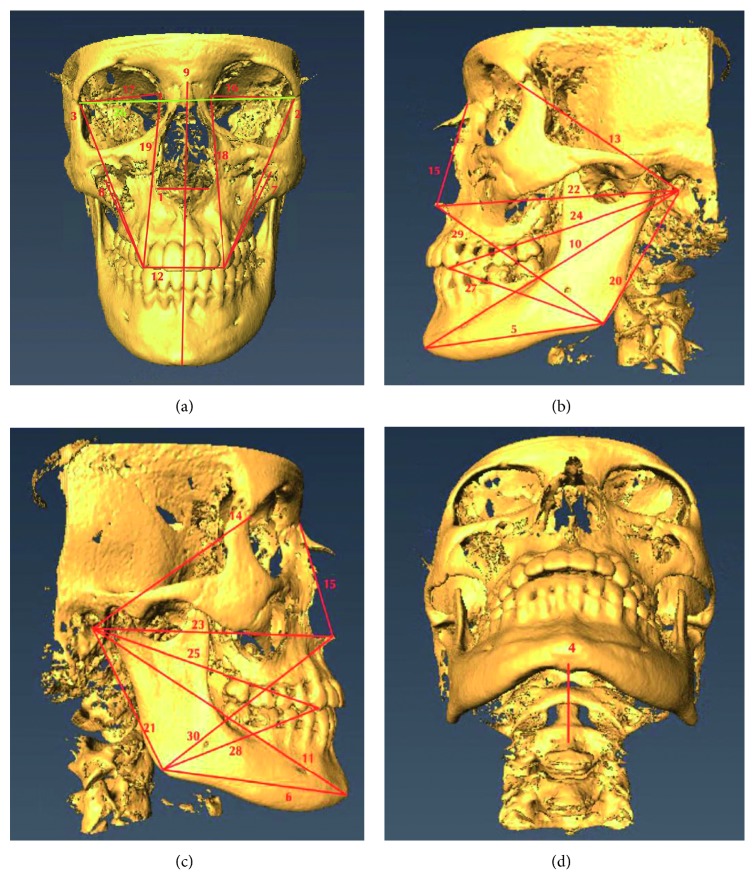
Front, left lateral profile, right lateral profile, and inferior views of the CBCT image, with numbered areas corresponding to landmarks. (a) Frontal view. (b) Left lateral profile view. (c) Right lateral profile view. (d) Inferior view.
